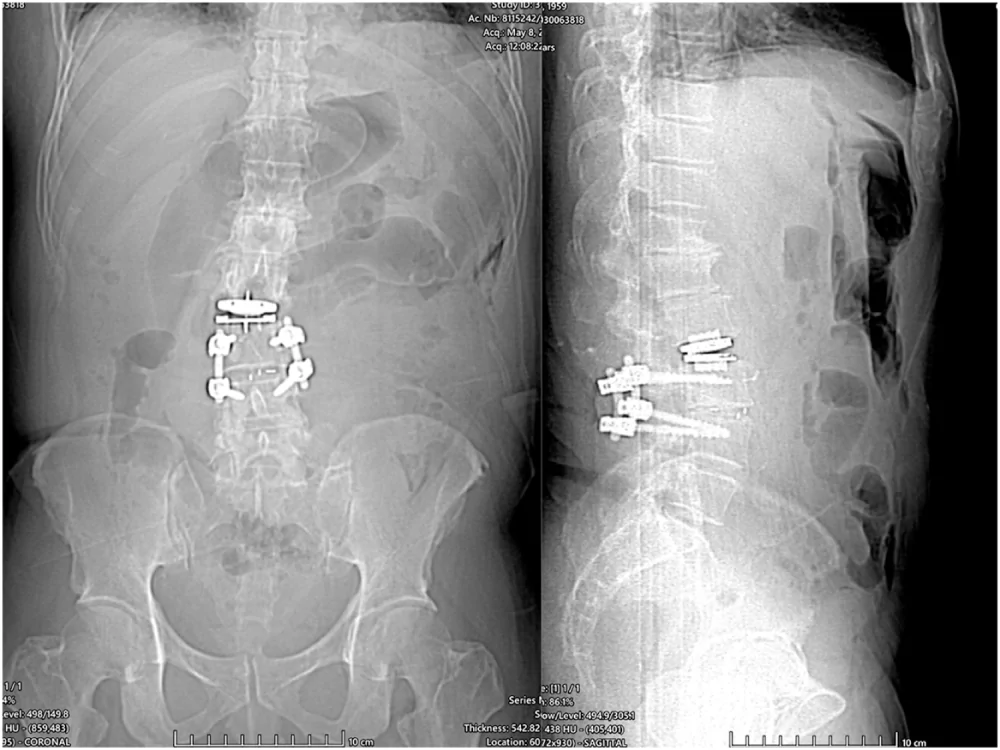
Postoperative X-ray showed L2–L3 lumbar disc replacement.
